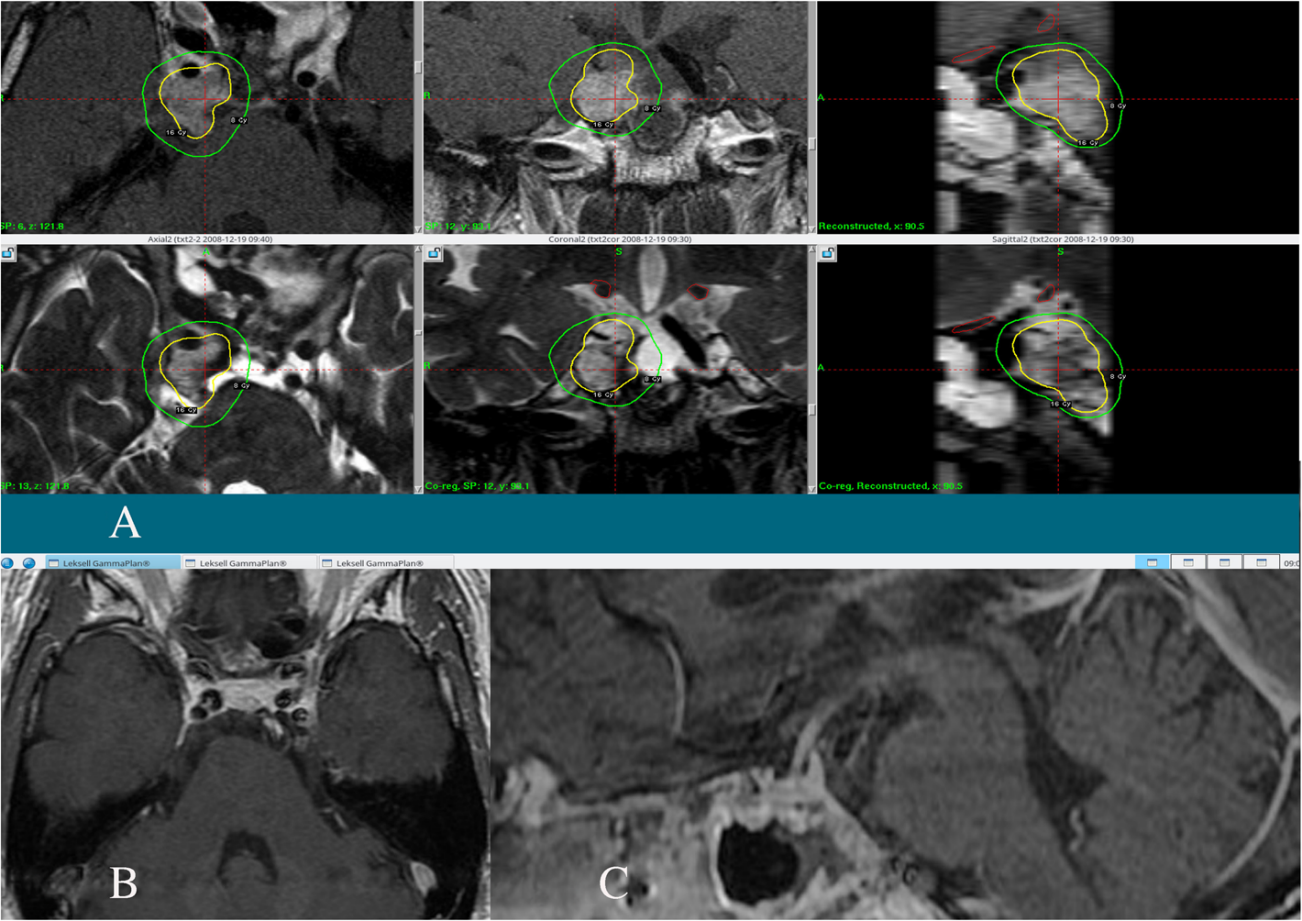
GK radiosurgery was planned with a marginal dose of 16 Gy. The yellow line indicating 16 Gy, and the green as 8 Gy a. Follow-up MR imaging revealed complete remission of the tumor (b: axial image, c: sagittal image)
